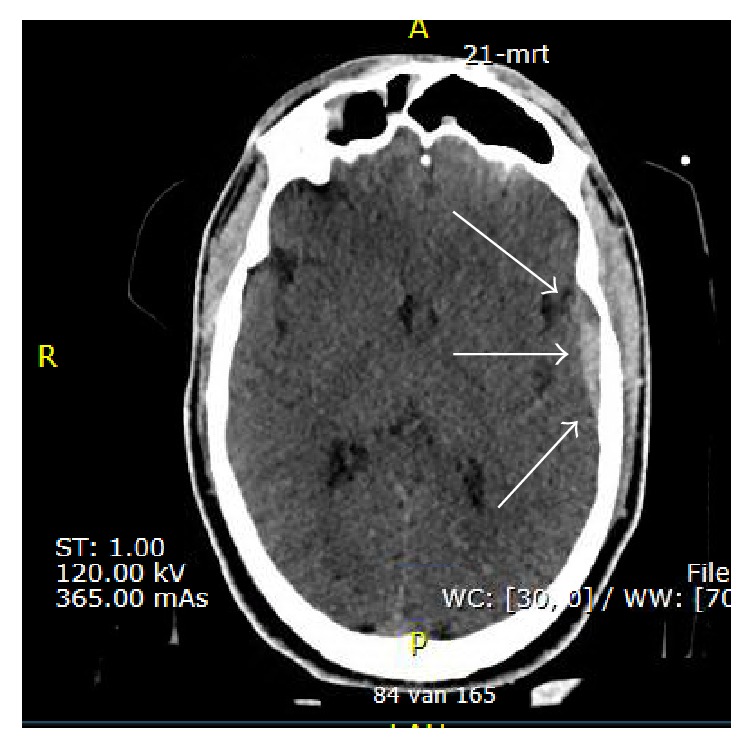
Cranial CT scan of a motor-cross rider, in which the prehospital Infrascanner measurement suggested an intracranial hematoma in the left parietal region. The cranial CT confirms this suspicion (white arrows). Cave: by convention, the CT image is left/right flipped, in respect to the Infrascanner image.
